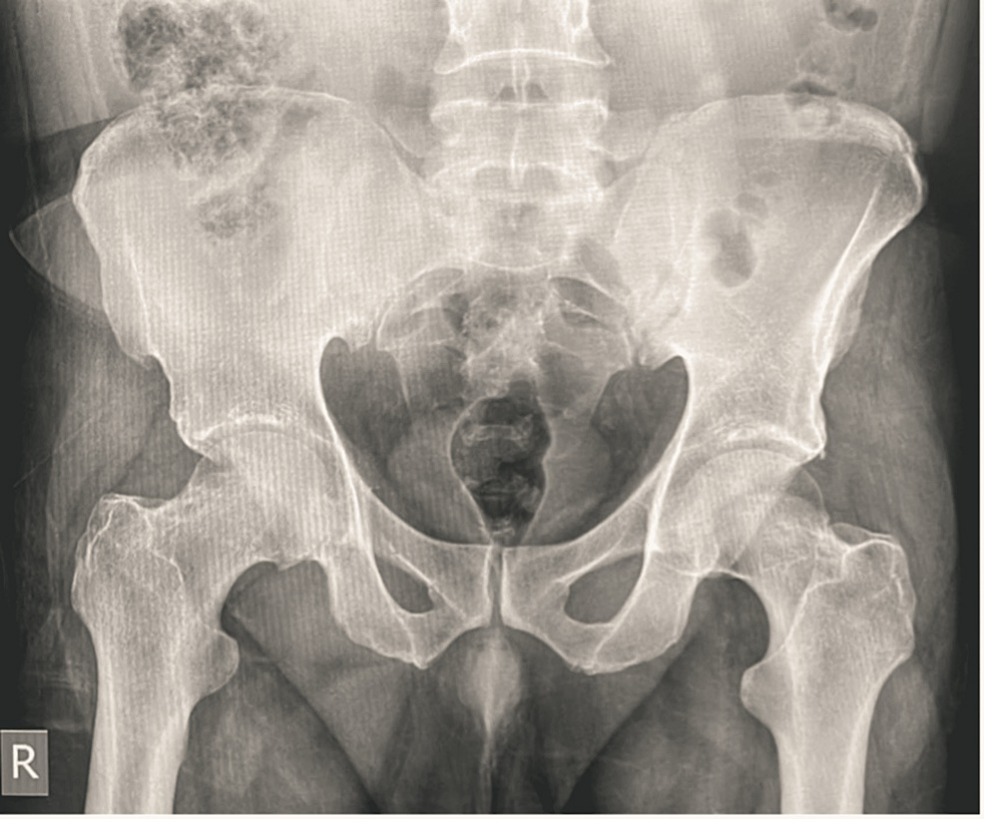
Pelvic x-ray at time of presentation.
